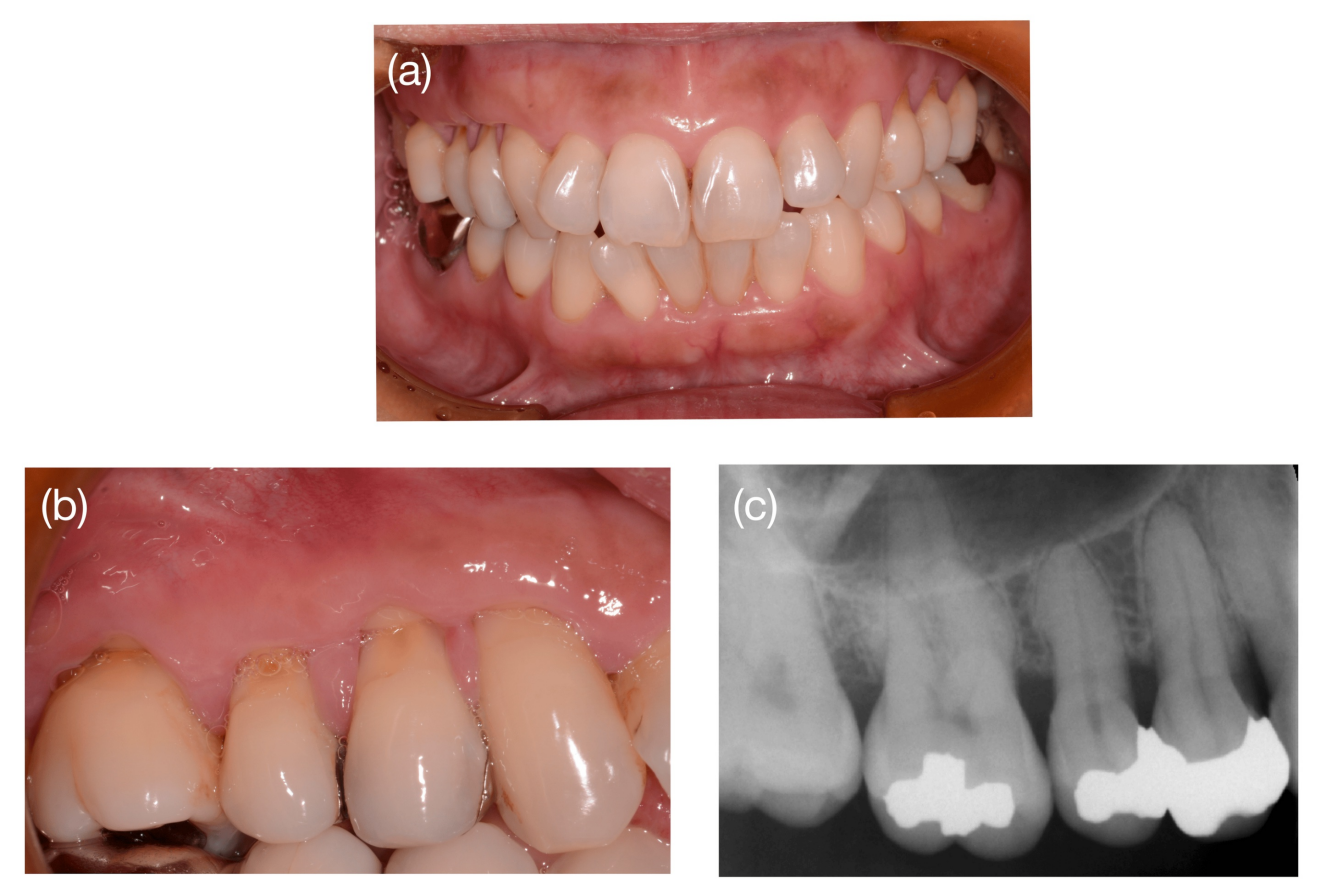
Initial views captured in a 47-year-old woman a) Facial view. b) Severe noncarious cervical lesions (NCCLs) were observed in the right maxillary region. c) Only mild bone resorption was confirmed, and no remarkable findings were noted on the radiograph.
